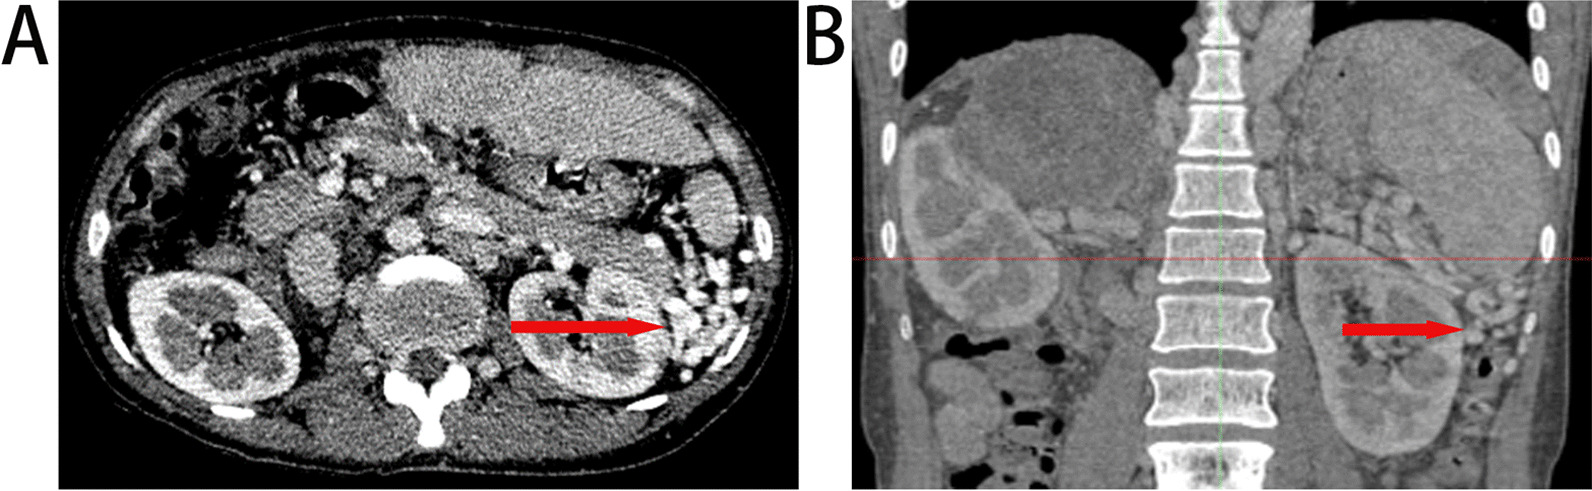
portal collateral vessels: varicose vessels located in the lower pole of the spleen: A Transverse CT image of the portal collateral vessels located in the lower pole of the spleen. B Coronary CT image of the portal collateral vessels located in the lower pole of the spleen
